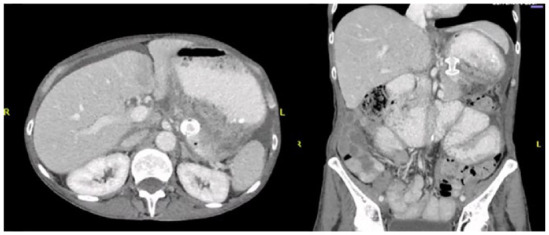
Axial and coronal abdominal CT images 1 week after EUS-guided transgastric placement of LAMS. EUS, endoscopic ultrasound.
